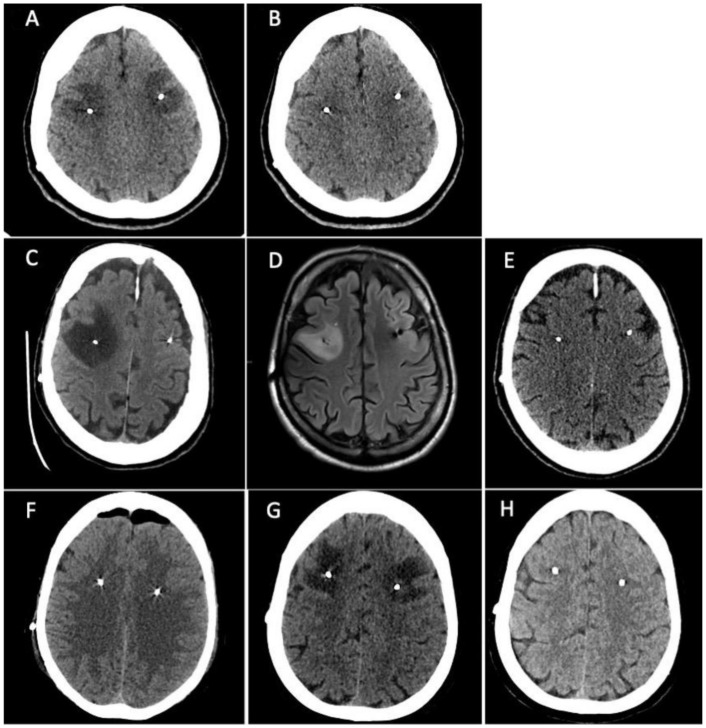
Patient 1. (A) Brain CT scan showed the presence of bilateral frontal subcortical edema surrounding the DBS electrodes. (B) a new brain CT scan showed the complete resolution of brain edema. Patient 2. (C) CT scan revealed the presence of a massive cerebral edema in right frontal lobe, surrounding DBS electrodes. (D) Brain MRI performed fourteen days later confirming the presence of a T2-weighted and FLAIR sequences hyperintense area in the right middle frontal subcortical lobe compatible with cerebral edema. (E) Brain CT scan repeated 30 days later showed the resolution of cerebral edema. Patient 3. (F) postoperative CT scan showed mild asymptomatic left frontal edema surrounding the homolateral DBS electrode. (G) Brain CT scan was repeated showing a great worsening of left middle-upper frontal edema that surrounded the DBS electrodes bilaterally, extending also to the left parietal lobe and near the right coronal suture. (H) Brain CT scan showed resorption of brain edema.
